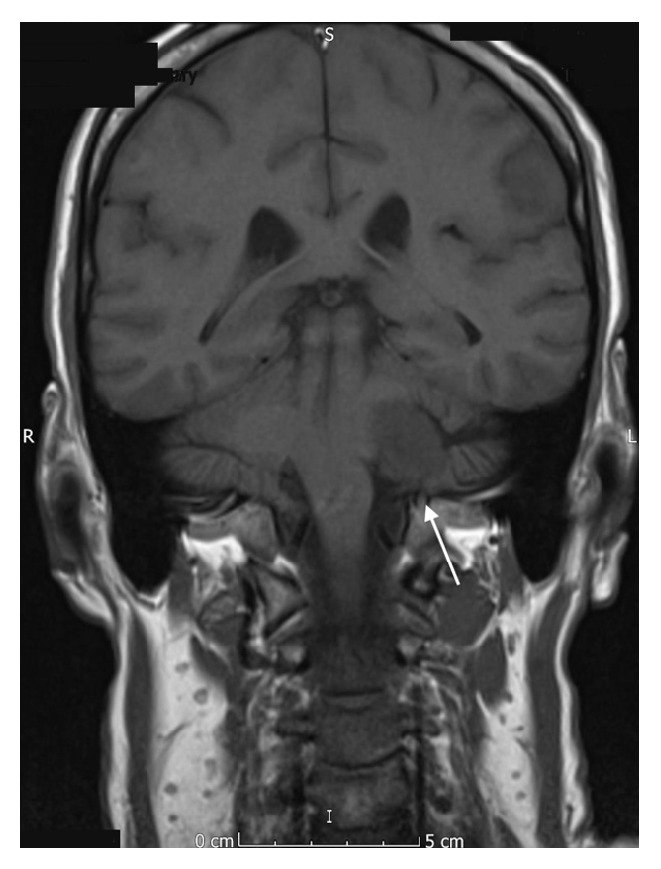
Coronal MR image (T1 weighted) showing the vestibular schwannoma affecting the left vestibulocochlear nerve.
